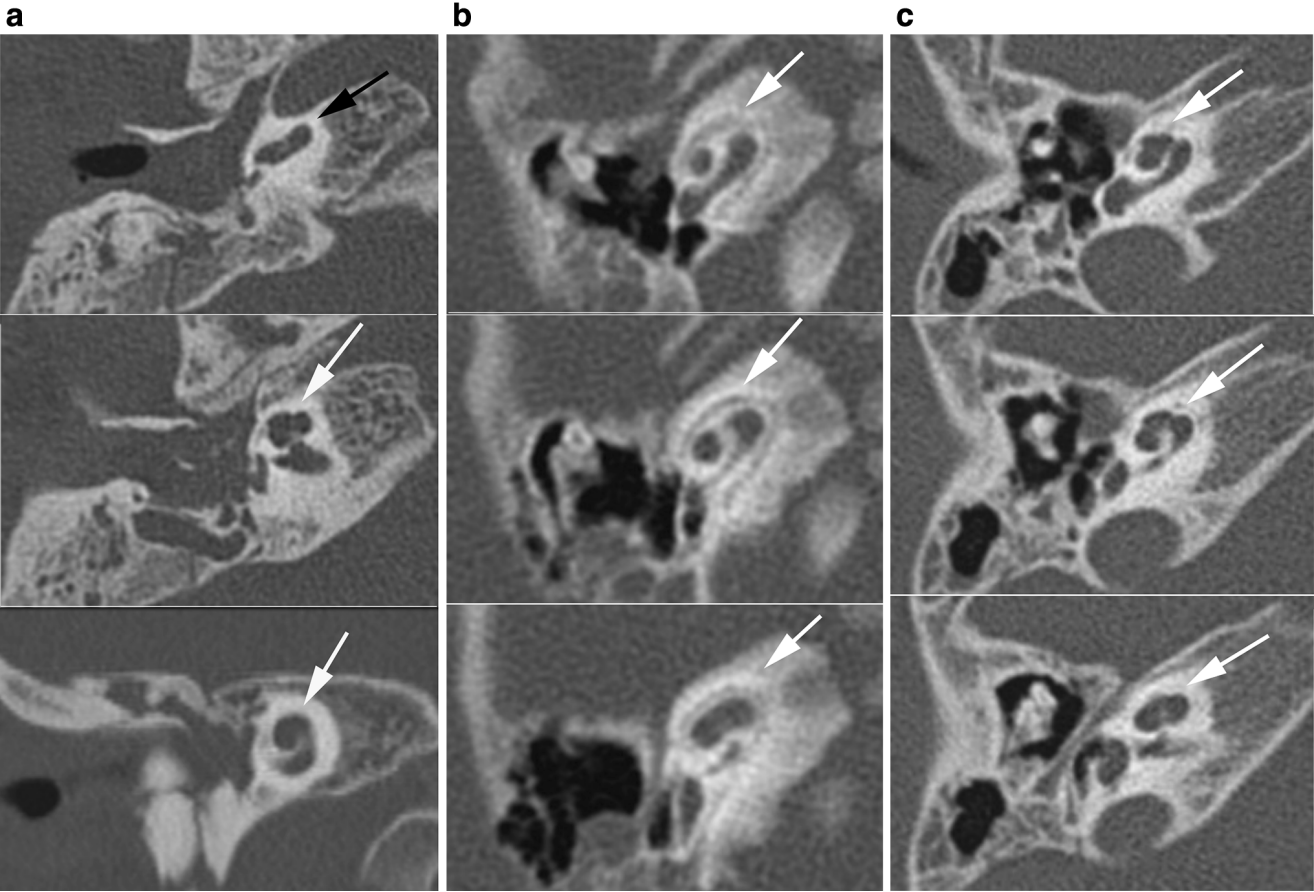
Range of abnormalities of the cochlea seen in axial CT images. a Incomplete partitioning type II: normal development of the basal turn, but fusion of the second and apical turn seen in axial and coronal planes. b Hypoplasia type III: cochlea with less than 2 turns. c Cochlea type ‘IV’: the basal, second and apical turns are present, but the second turn seems shortened, giving the cochlea an asymmetric, flattened appearance
